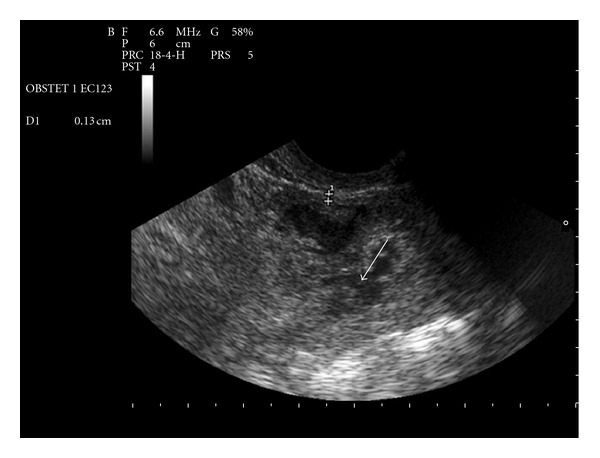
Transvaginal ultrasound image of the scar pregnancy only 1.3 mm of myometrium visualized in the anterior wall of the cervix, empty cervical canal (arrow).
